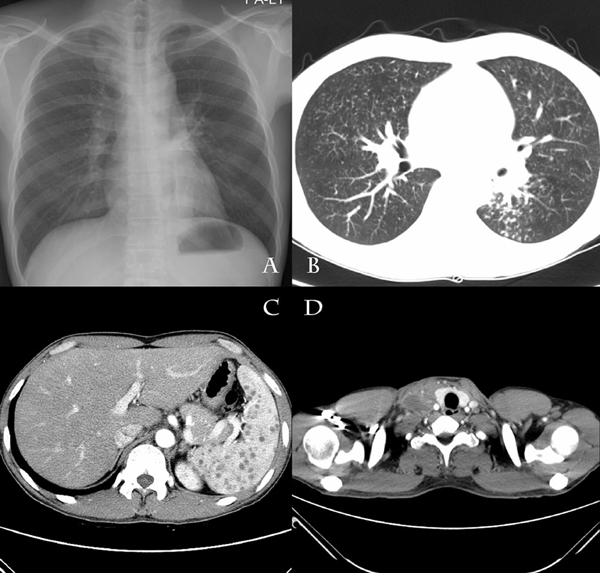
Chest X-ray and computerized tomography scans of the abdomen and neck.
